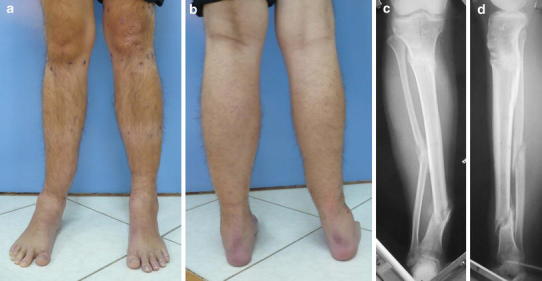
a, b preoperative clinical photos show angular deformity of the lower leg due to fracture of a lengthening regenerate; c, d preoperative x-rays show hypertrophic nonunion of the lower third of the tibia with medial angulation
